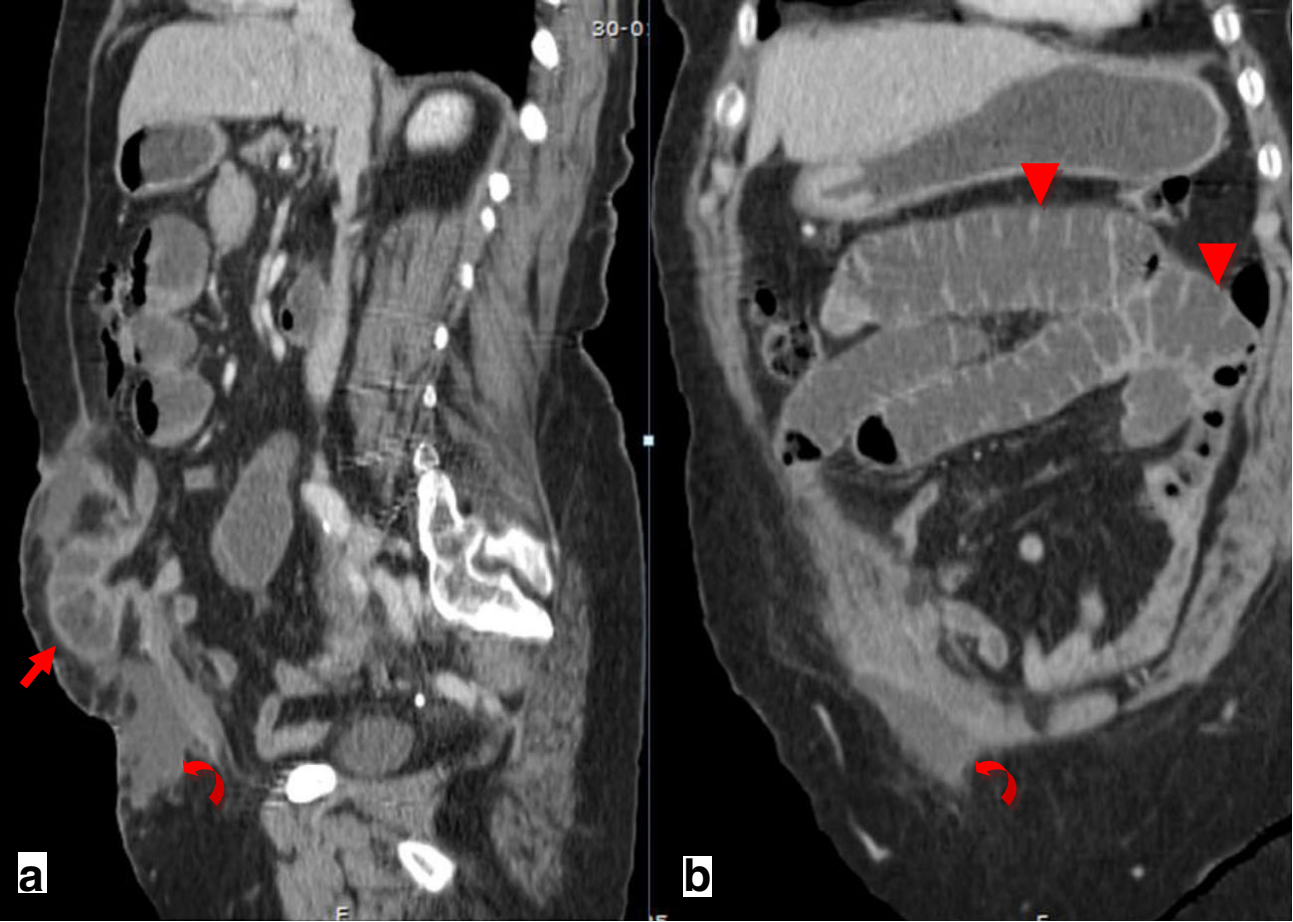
a, b Abdominal CT of a patient with obstructive symptoms. There is diffuse fluid dilation of small-bowel loops (*arrowheads in b*) proximal to a small segment of dilated small bowel incarcerated at the hernial sac (*arrow in a*). The presence of fat stranding and a moderate amount of free fluid at the site (*curved arrows in a, b*) should raise suspicion of a strangulated hernia
